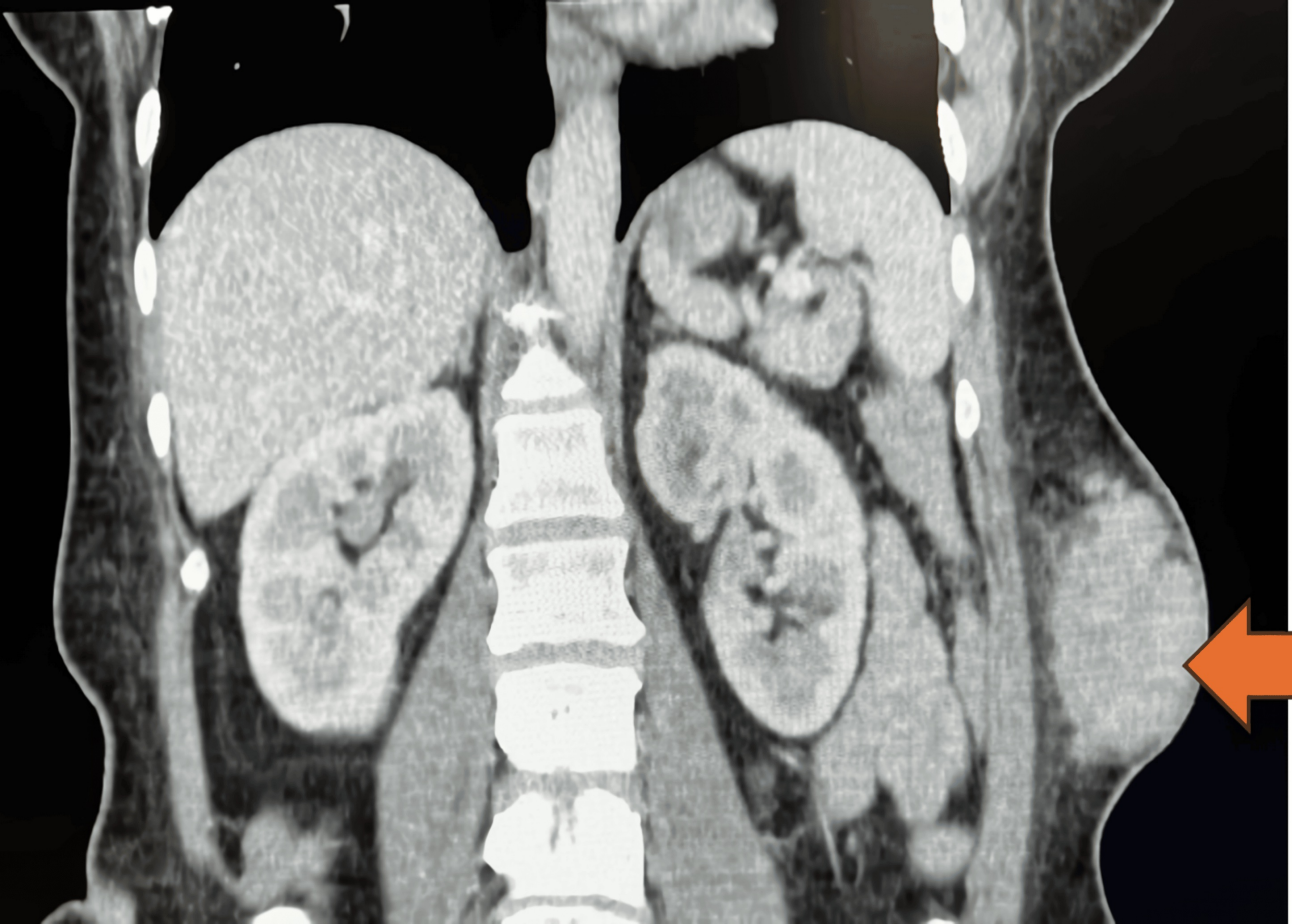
CT of abdomen (sagittal view): The orange arrow indicates the soft tissue mass on left flank
